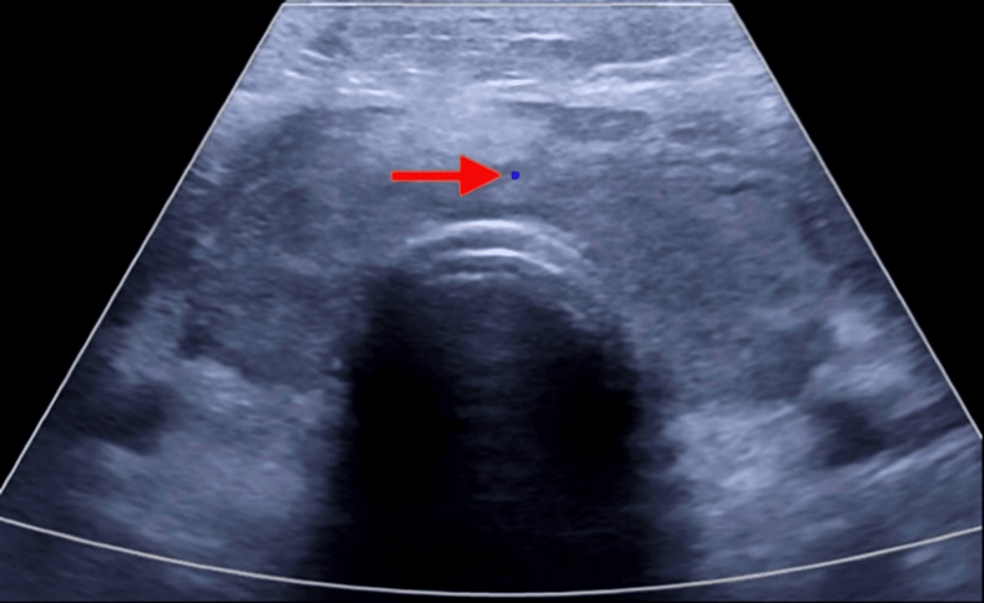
Color flow ultrasound of the thyroid gland, demonstrating a heterogeneously enlarged thyroid with reduced vascularity (red arrow).
